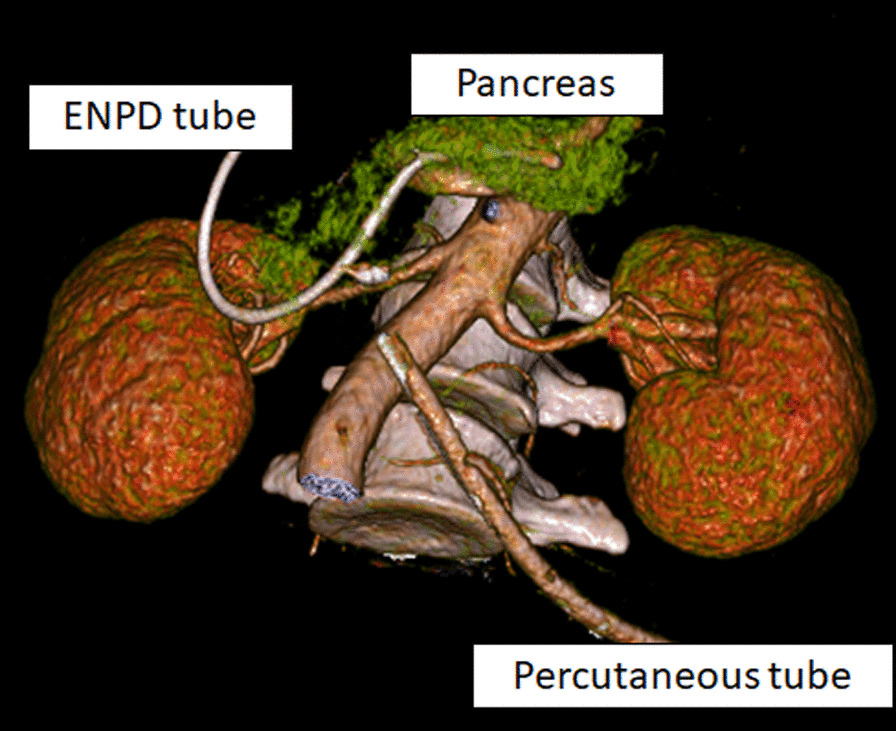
3-Dimensional image of the abdominal computed tomography scan showing the stent across the ductal disruption and percutaneous drainage tube.
